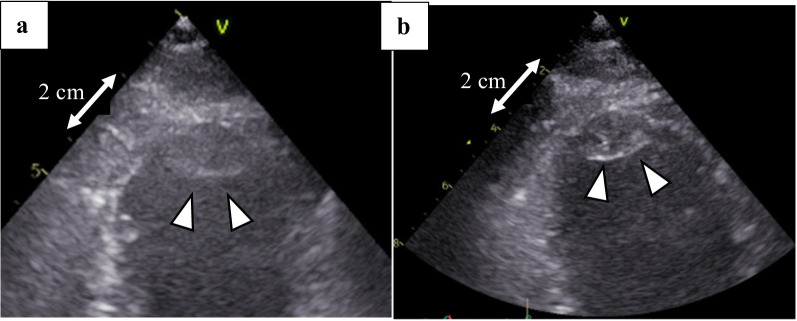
Postoperative echocardiography with intracardiac thrombus (white arrows). a: 7 days after the surgery. b: 1 month after surgery
